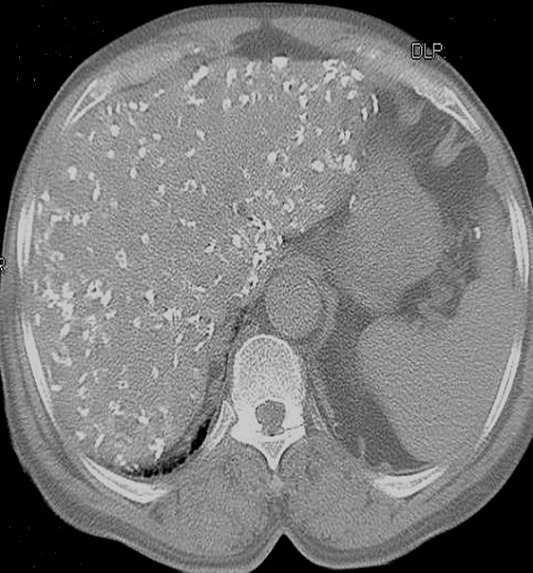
Non-enhanced axial CT-slice of the abdomen at the level of the liver (Bone window) showing multiple hyperdense lesions of calcific density in the liver, with parenchymal distortion; calcific deposits are also noted on the splenic capsule and bowel wall
